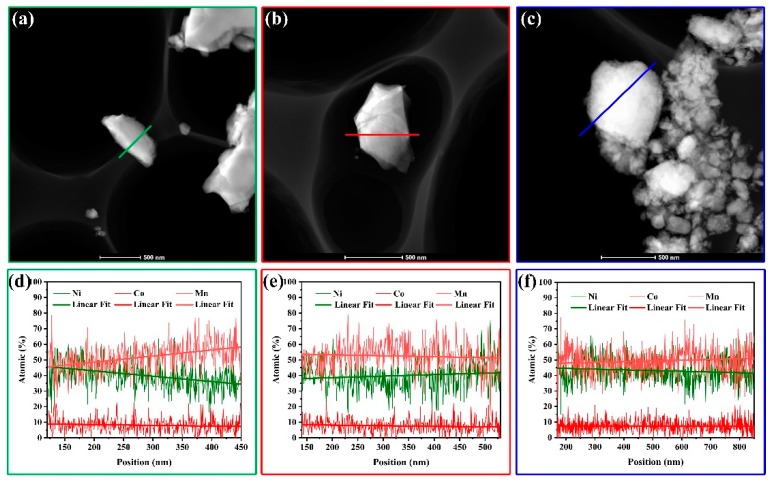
TEM images and X-ray line scan element distribution (EDX) maps: (a,d) SLC; (b,e) SLS; (c,f) OCP.
